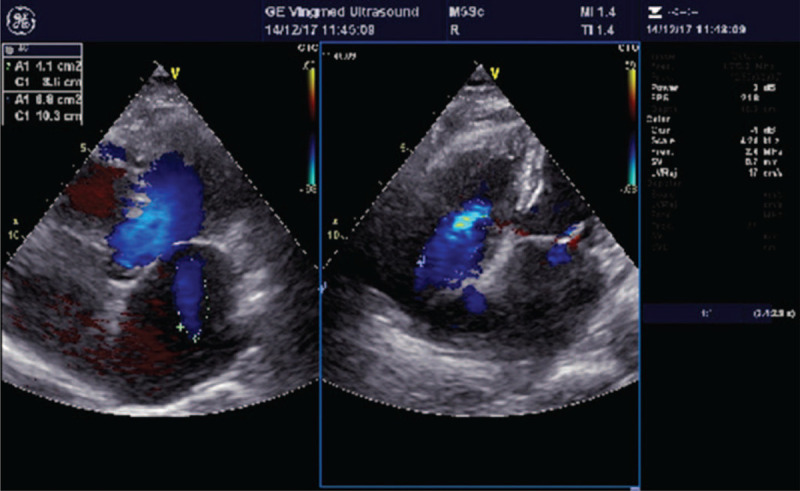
Echocardiography showing no thrombus in the left atrial appendage after 50 days of anticoagulation with warfarin.
